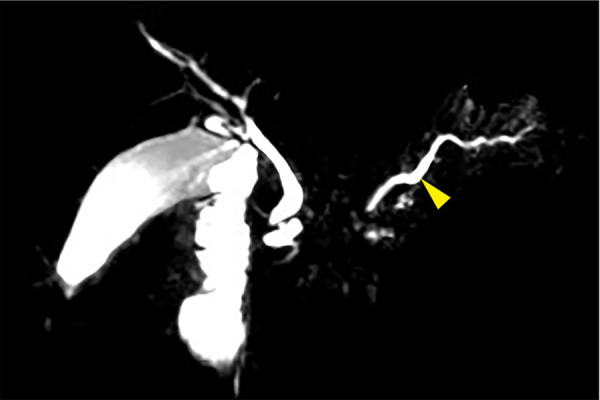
(Case 2): follow-up MRI/MRCP 25 months postoperatively shows mild local dilation of the pancreatic duct, with a maximum width of approximately 2.9 mm (yellow arrowhead).
